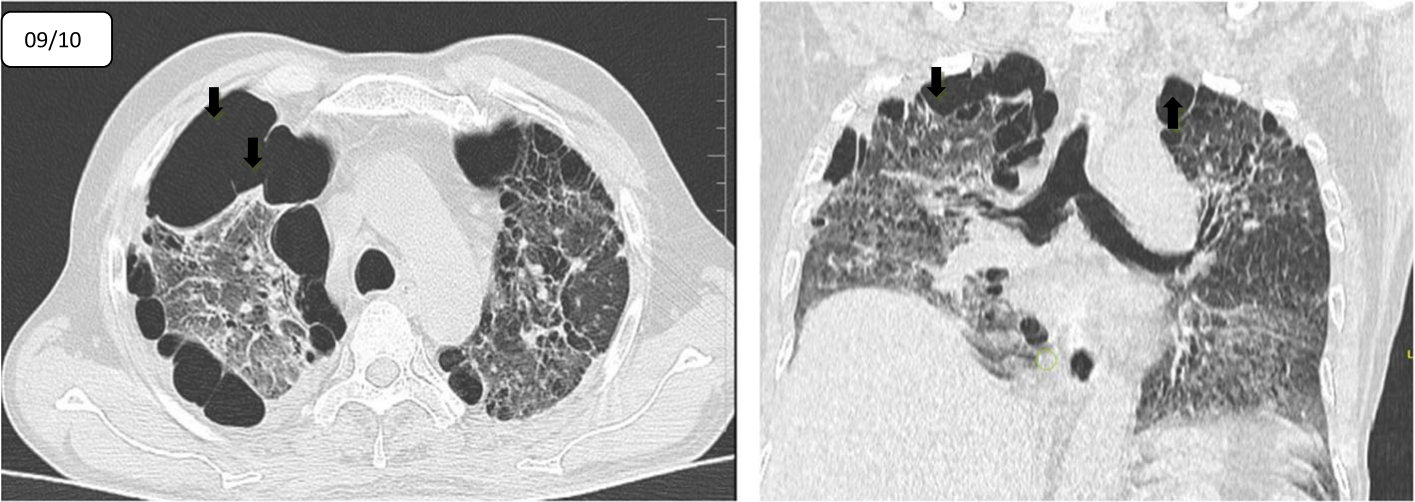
a, b Repeat HRCT after 1 month axial and coronal sections shows marked increase in emphysematous and bullous changes (black arrow) with reticular thickening and ground glass opacities
